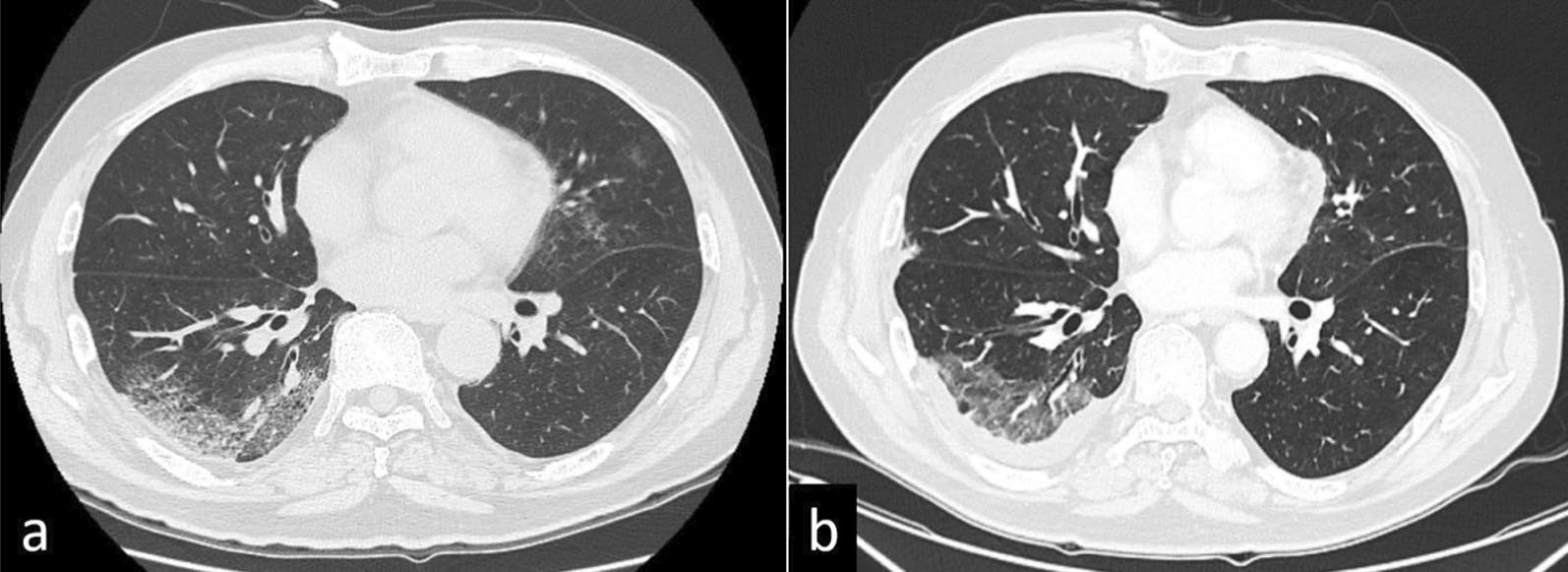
Lung findings of case 1 on chest computed tomography. a Peripheral ground-glass opacities and band-like opacities were found in bilateral lower lobes immediately after COVID-19 infection. b The ground-glass opacities regressed 23 days before the operation
